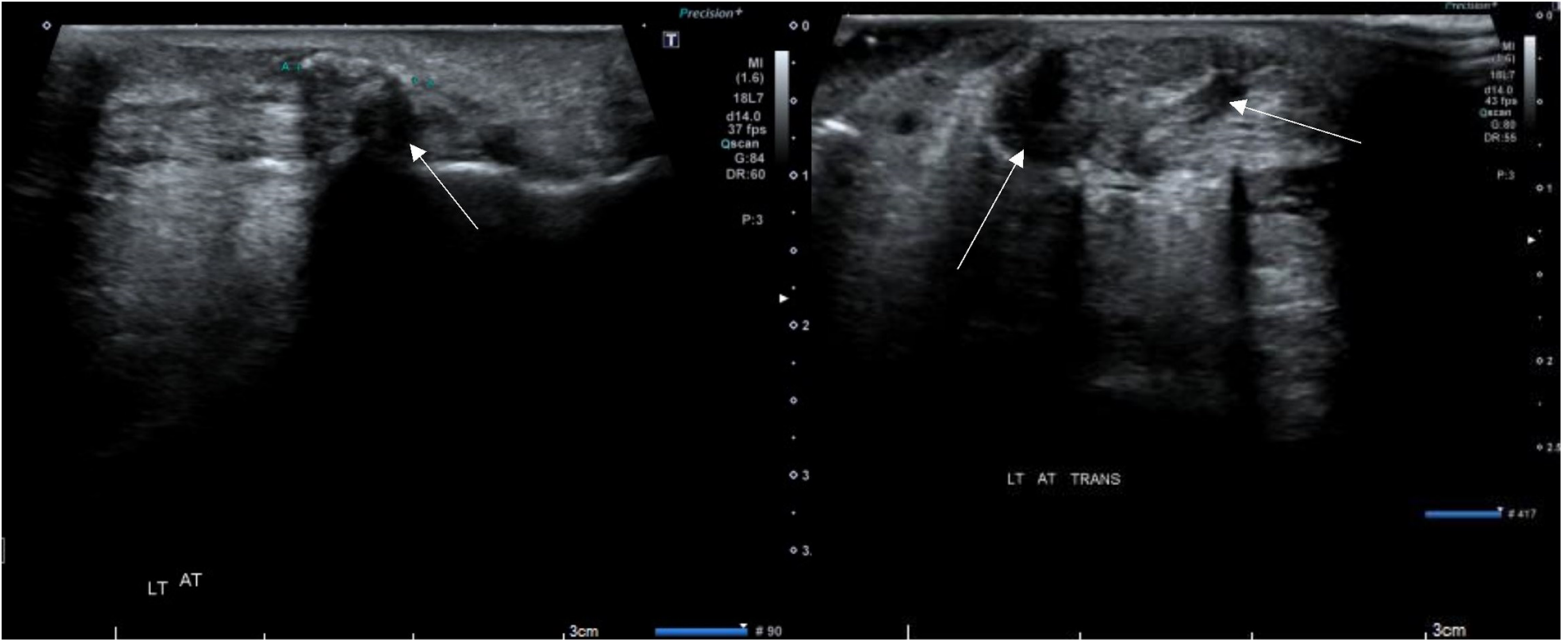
Ultrasonography images of the patient's AT after the first surgery. The sagittal image (left) shows an increase in mucoid and fluid content, indicating post-operative inflammation. The transverse image (right) displays fluid accumulation surrounding the AT, with bright spots enhancing the image.
